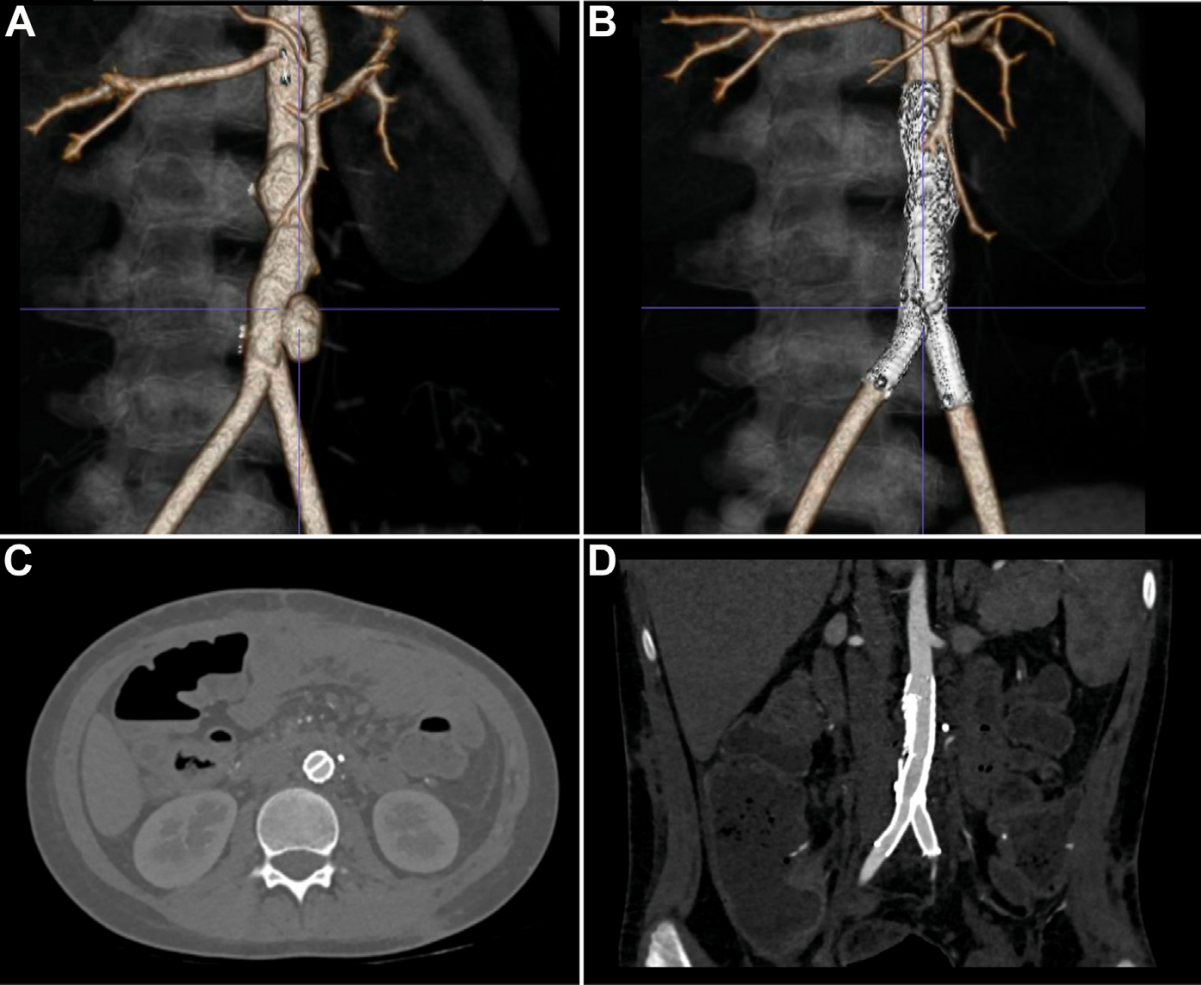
A, Three-dimensional reconstruction of computed tomography (CT) angiogram demonstrating the aortic pseudoaneurysm before intervention. B, Three-dimensional reconstruction of CT angiogram at the 6-month postoperative visit showing patent endografts with complete resolution of the pseudoaneurysm. C, Axial view of postoperative CT angiogram demonstrating complete obliteration of the gutters between the endografts. D, Coronal view of postoperative CT angiogram showing complete resolution of the pseudoaneurysm.
